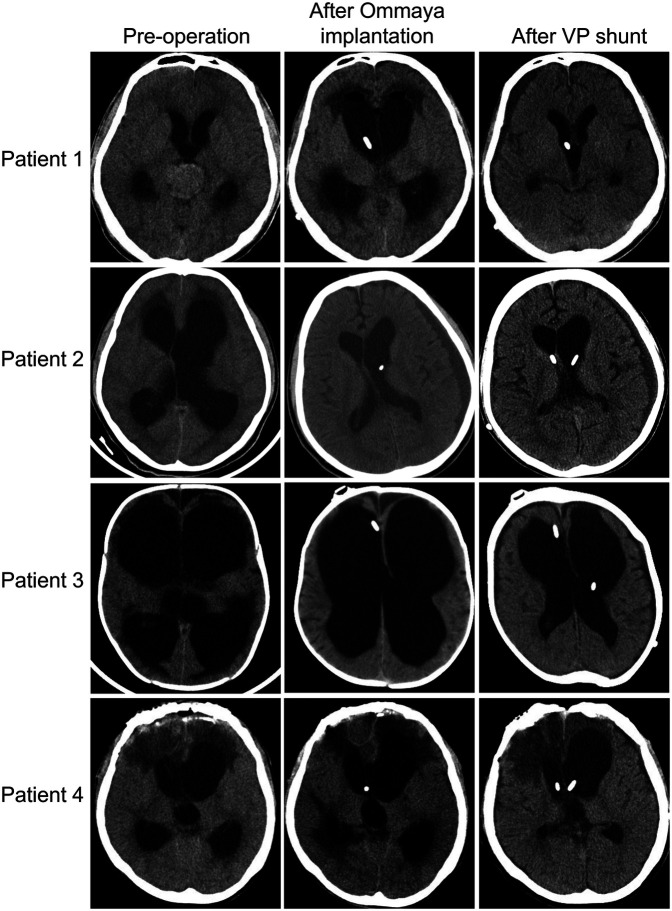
Cranial computed tomography images of four patients with L&NPH before hydrocephalus surgery, after Ommaya capsule implantation and after lateral VPS.
